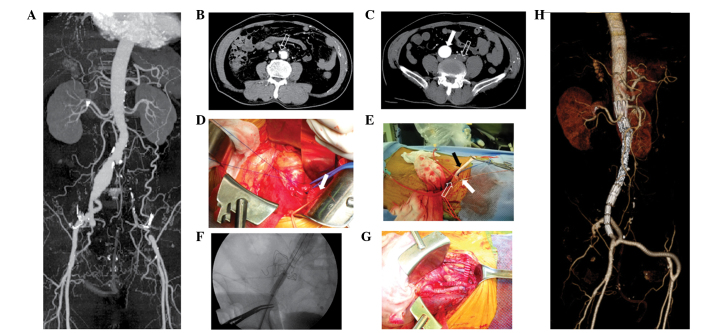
Case 1. A 70-year-old male patient had (A) a right common iliac artery (CIA) aneurysm, long-segment occlusion of the left iliac artery and (B) a penetrating atherosclerotic ulcer in the infrarenal abdominal aorta (indicated by the open arrow). (C) The axial image shows the CIA aneurysm (white arrow) and the occluded left iliac artery (white arrow). (D) The external iliac artery (EIA) was tortuous at the time of exposure. (E) Two introducer sheaths were inserted into the right EIA for angiograms (closed arrow) and for the delivery of the stent graft (empty arrow) with separate punctures. The anastomosed graft was seen in this picture (black arrow). (F) Endovascular repair of the right CIA aneurysm was successful. (G) External-to-internal iliac artery bypass, end-to-end anastomosis following the division of the tortuous EIA and extra-anatomic crossover femoral bypass were performed. (H) Postoperative computed tomography-angiography showed that the repair of the aneurysm and the patent bypassed graft was successful.
